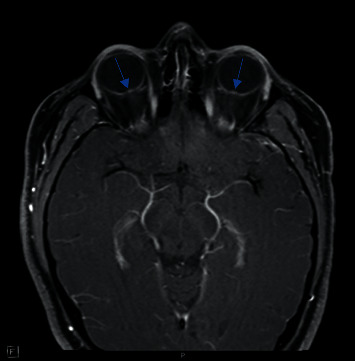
MRI brain. Dilated CSF space is visualised surrounding the optic nerves bilaterally. Possible bilateral optic nerves protrusion into the vitreous space.
